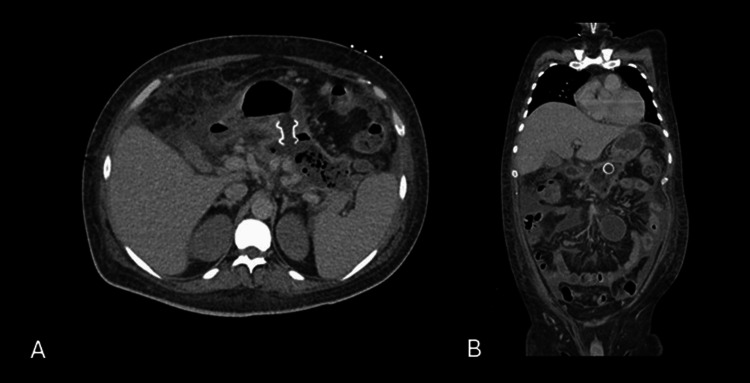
CT progression of necrotizing pancreatitis post-ETD. Post-first endoscopic transluminal drainage (ETD), axial (A) and coronal (B) CT images show a lumen-apposing metal stent (LAMS) placed through the posterior gastric wall into the necrotic collection, with a marked reduction in collection size.
